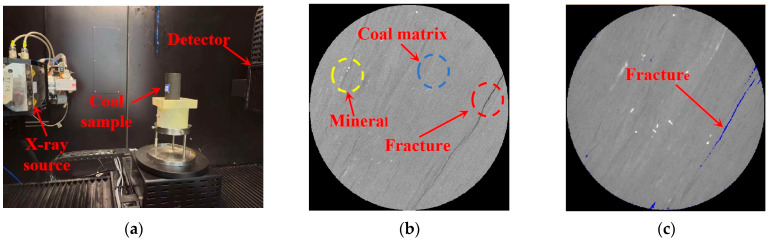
Fissure extraction process: (a) CT scan; (b) 2D slice; (c) threshold segmentation.
